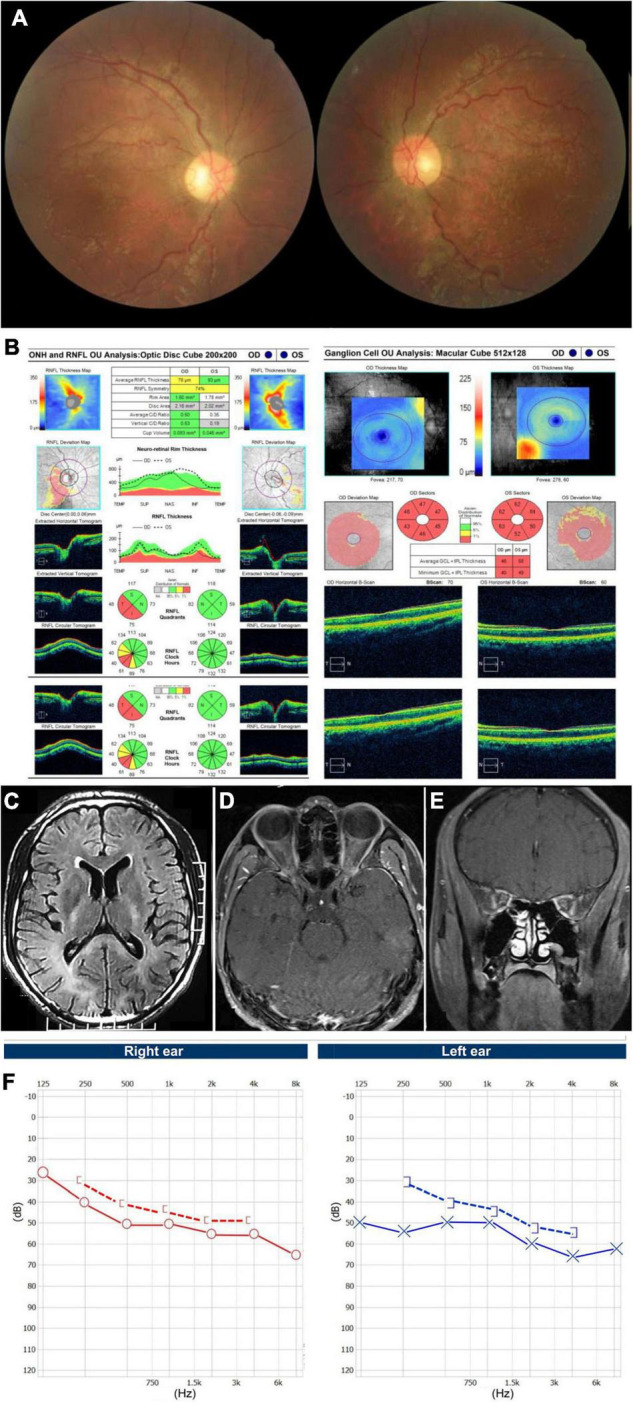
Accessory examinations of case 1. (A) Fundus photos showed diffuse pallor in the right eye and temporal pallor in the left eye, with tortuous retinal vein bilaterally. (B) OCT showed thinning of inferior-temporal RNFL in the right eye and severe GCIPL loss bilaterally. (C) Axial T2 Flair-weighted MRI of the brain showed hyperintense signal changes involving the posterior limbs of internal capsule, occipital lobes and corpus callosum indicating leukoencephalopathy. (D) Axial and (E) coronal T1-weighted MRI of orbits with gadolinium and fat suppression showed bilateral optic nerve slight enhancement. (F) Audiography showed bilateral neurosensory deafness.
